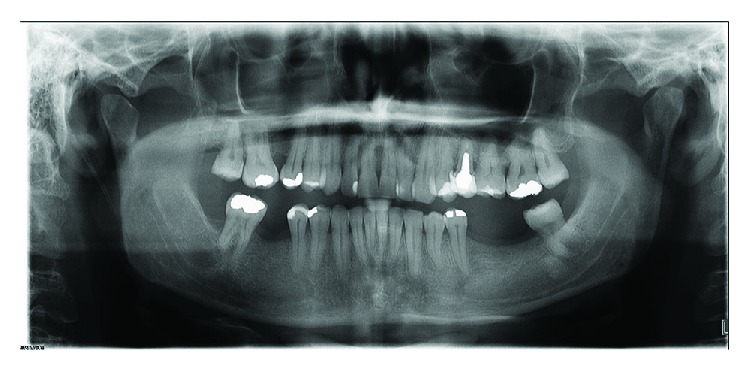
Patient's orthopantomogram (OPG) showing bone healing after a 2-year follow-up.
